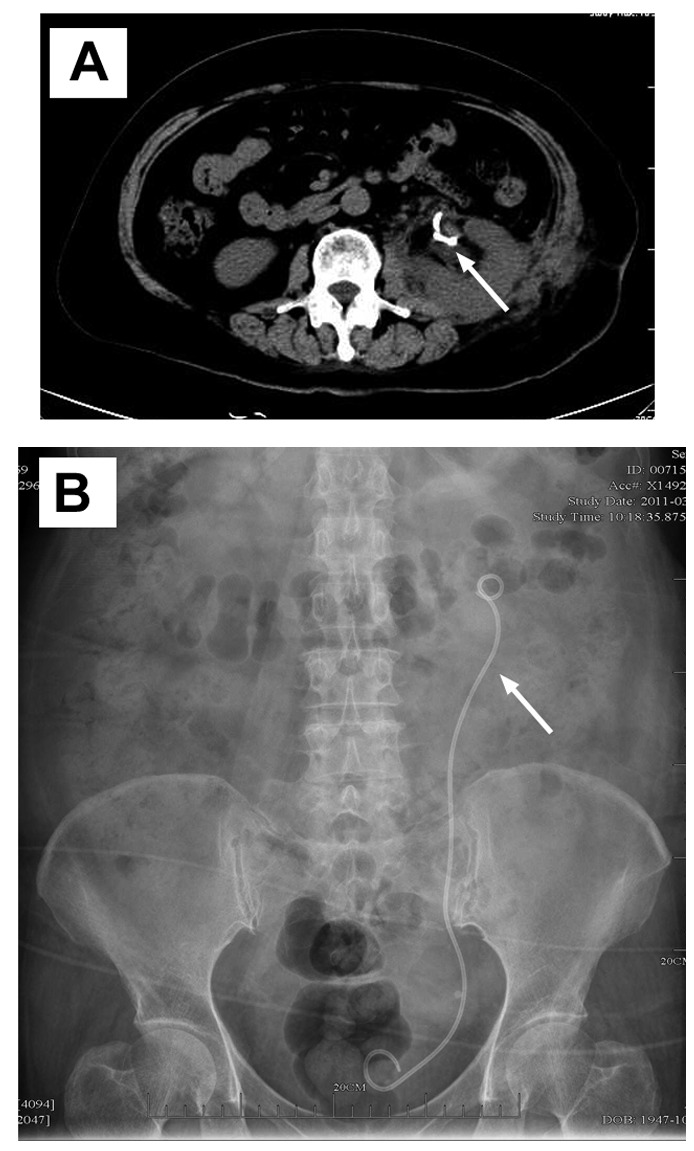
Postoperative (A) CT and (B) plain abdominal X-ray showing the double-J tube (arrow) in the correct position.
